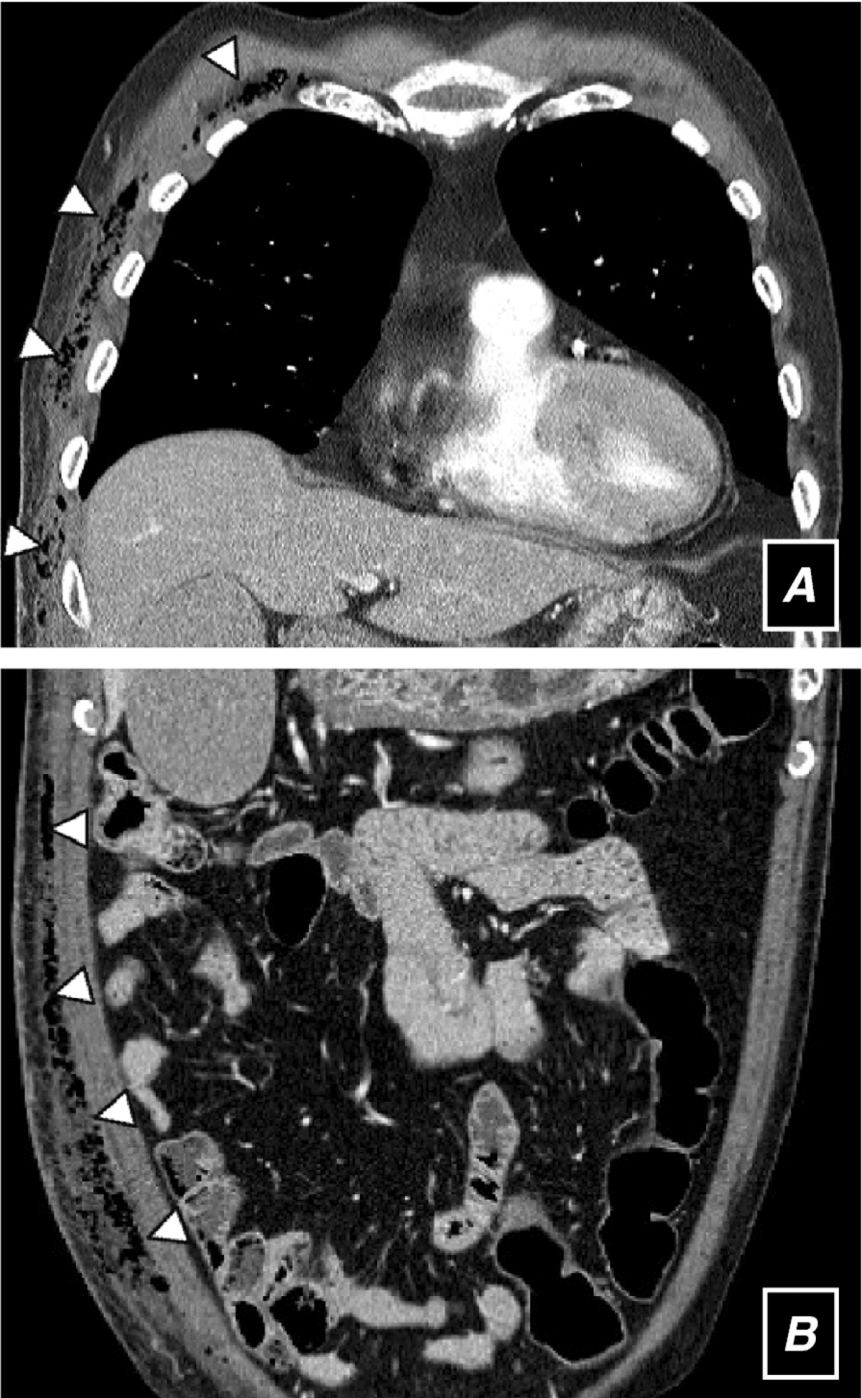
CT scans of the chest (a) and abdomen (b) show soft tissue edema, subcutaneous fat infiltrations extending along the fascial plane, and muscular thickening with gas in the right anterolateral aspect of the chest and abdominal wall (*arrowheads*).
